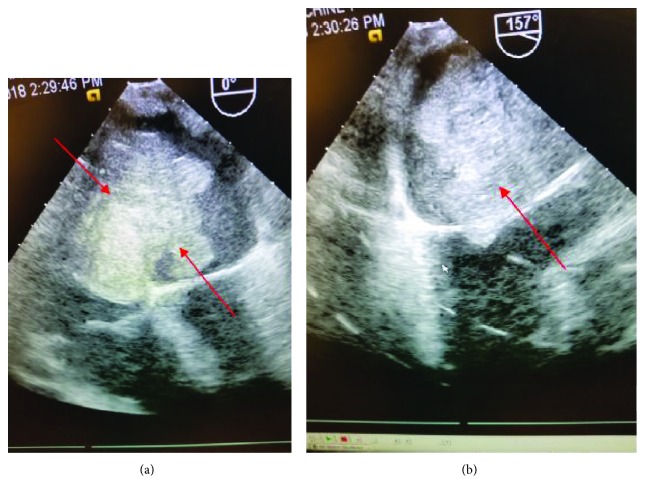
Transesophageal echocardiogram (intraoperative) demonstrating the mass in the left atrium invading the interatrial septum.
